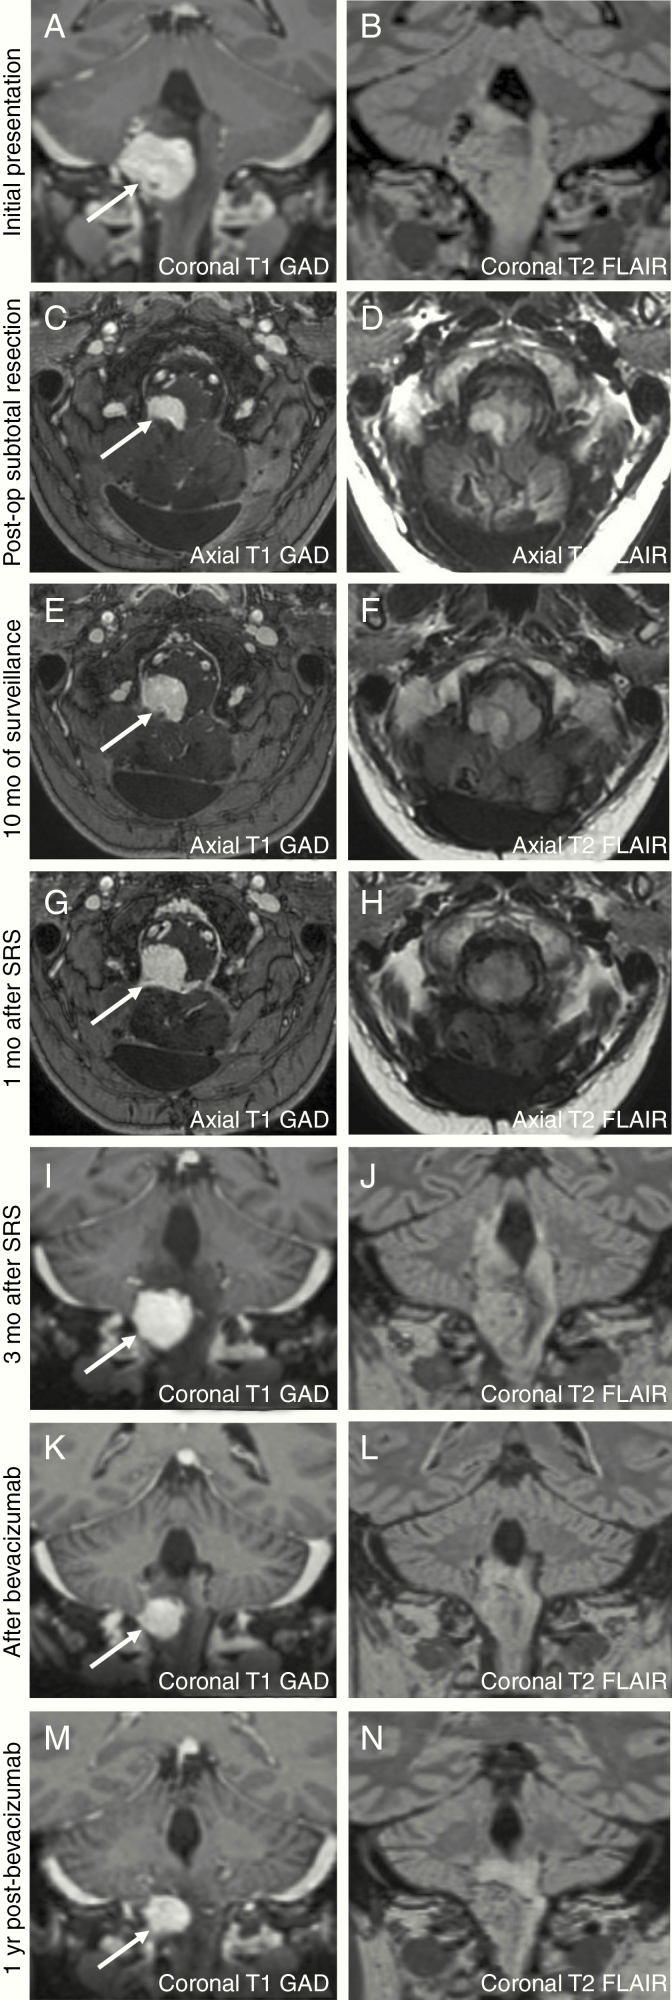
At the initial presentation, MRI revealed a mass at the cervicomedullary junction measuring 2 × 2.5 × 2.3 cm (A and B). After subtotal surgical resection (C and D), the size of the hemangioblastoma decreased to 1.6 × 0.6 × 1.1 cm. With 10 months of surveillance, the hemangioblastoma increased to 1.6 × 1.0 × 1.4 cm (E and F). There was an enlargement of the hemangioblastoma 1-month (mo) post-stereotactic radiosurgery, measuring 1.9 × 1.5 × 1.8 cm, with associated edema and hydrocephalus (G and H). Ongoing surveillance revealed a further increase of the hemangioblastoma (2.0 × 1.8 × 2.0 cm) and increased surrounding edema (I and J). After bevacizumab therapy, there was a decrease in the size of the hemangioblastoma, measuring 1.4 × 1.5 × 1.7 cm (K and L) and reduction of surrounding edema. Over 1 year of clinical surveillance, the hemangioblastoma has remained stable in size, measuring 1.4 × 1.6 × 1.7 cm (M and N).
